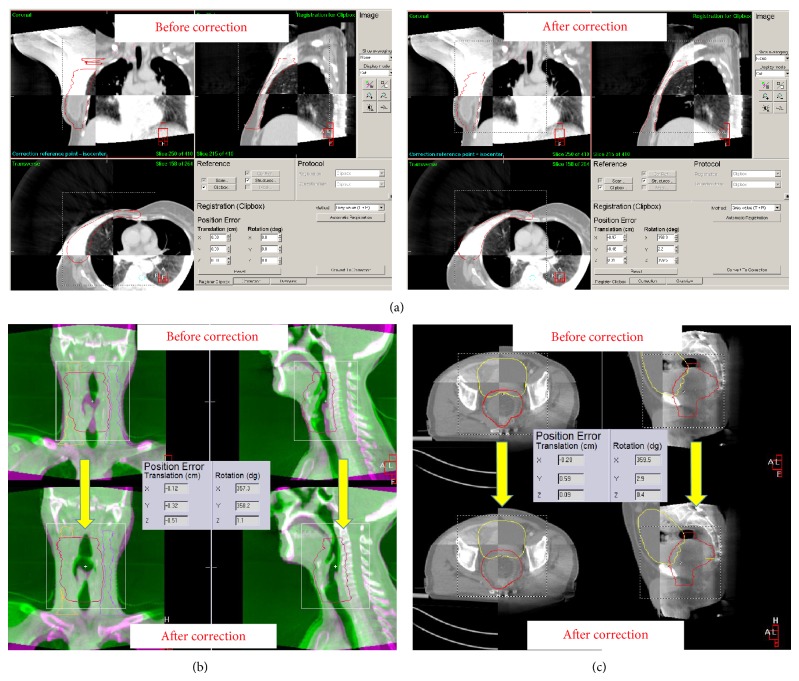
KV CBCT volumetric imaging. Both translational and rotational errors may be estimated. Translational errors are easily corrected whereas few systems have provisions for correcting rotational errors with couch rotations. (a) CBCT compared with reference scan before and after correction of setup error in a case of Carcinoma right breast, post-mastectomy. (b) CBCT correction in a case of Carcinoma larynx. (c) CBCT in a case of Carcinoma prostate not only corrects for setup errors, but also provides an estimate of reproducibility of prostate position with respect to bladder filling. In this particular case, the live image shows negligible bladder filling and treatment was delayed to allow for optimum bladder position for obtaining a reproducible position of prostate as well as moving the bowel out of treatment field. KV: kilovoltage; CBCT: cone beam computed tomography.
